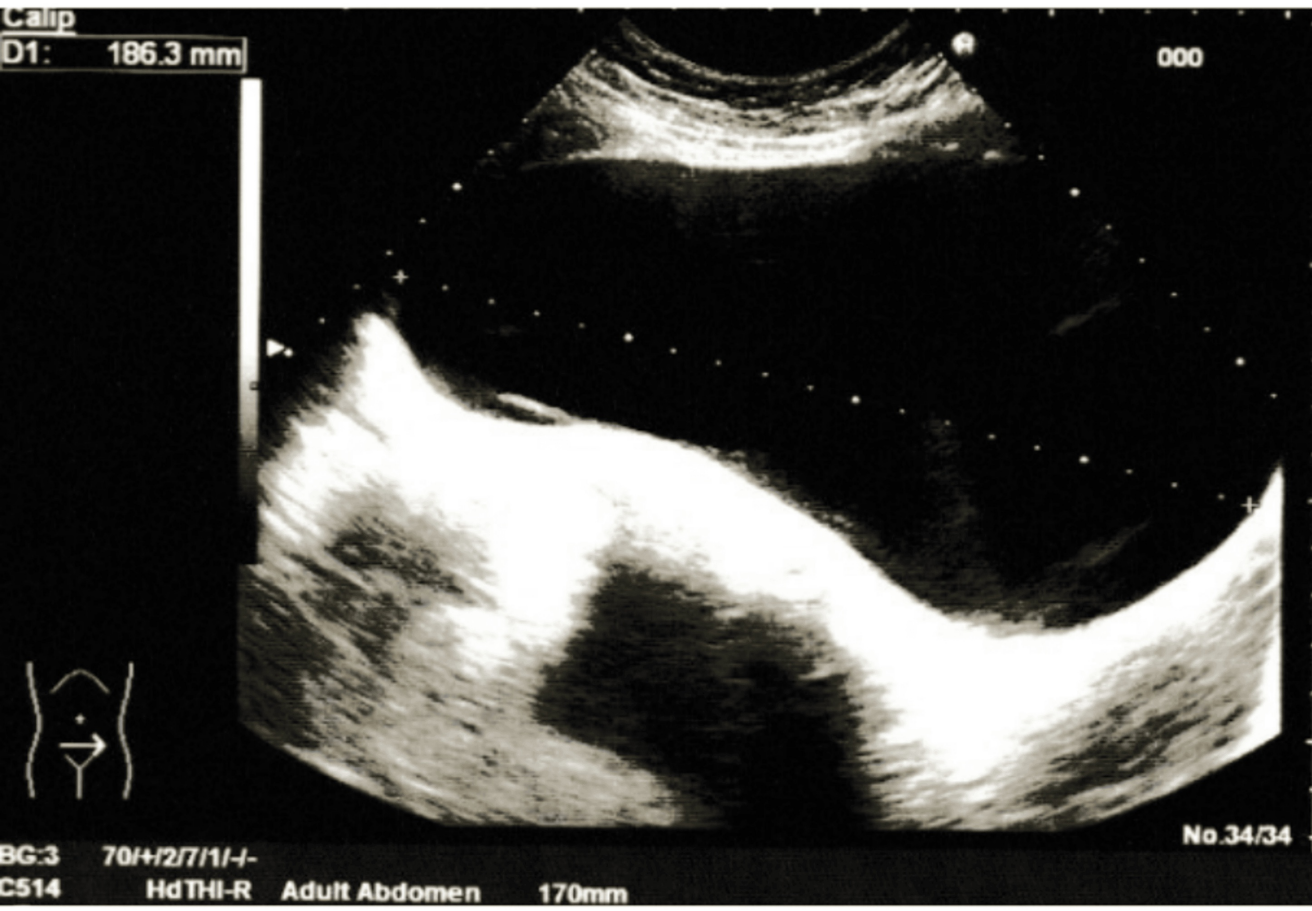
Pelvic ultrasound The image reveals a mass initially interpreted as a “distended bladder.”
